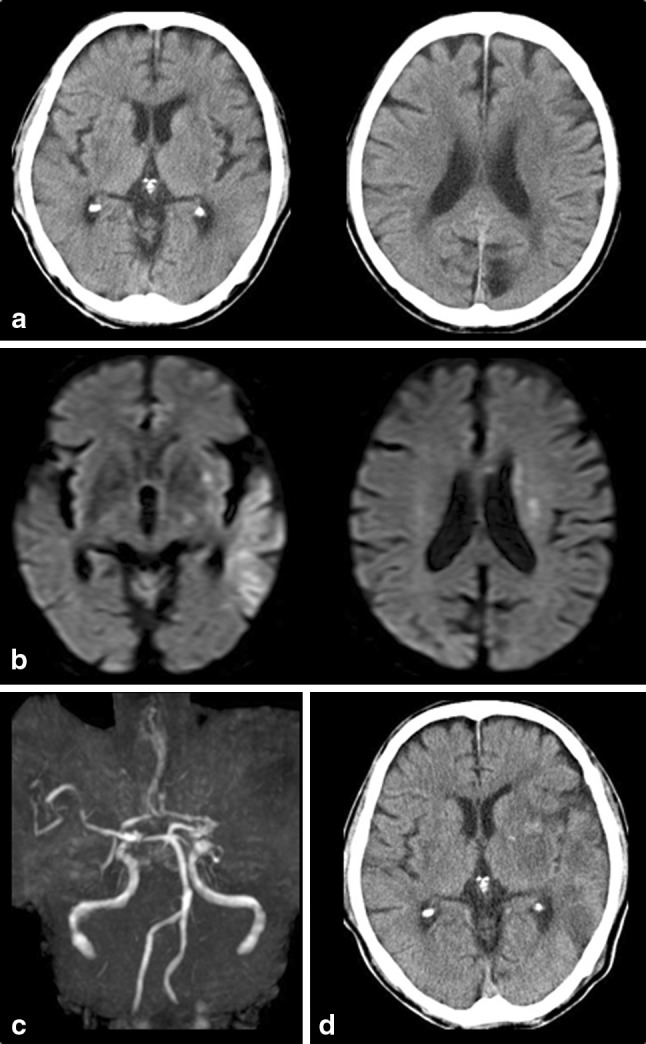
Case 1: 85-year-old man. Initial CT at 131 min of onset shows early ischemic change in the left L, I, and M3 (CT-ASPECTS, 7) (a). Initial DWI at 137 min of onset shows the hyperintense lesion in the left L, I, M2, M3, and W (DWI-ASPECTS, six and ASPECTS+W, six) (b). MRA on admission (c) shows occlusion of the left M1 proximal portion. The NIHSS score before IV-tPA was 19. IV-tPA was started 176 min of onset. The NIHSS score after 24 h was 15. The patient had asymptomatic ICH (HI 1) 24 h after tPA injection (d). The modified Rankin scale score at 90 days was three
